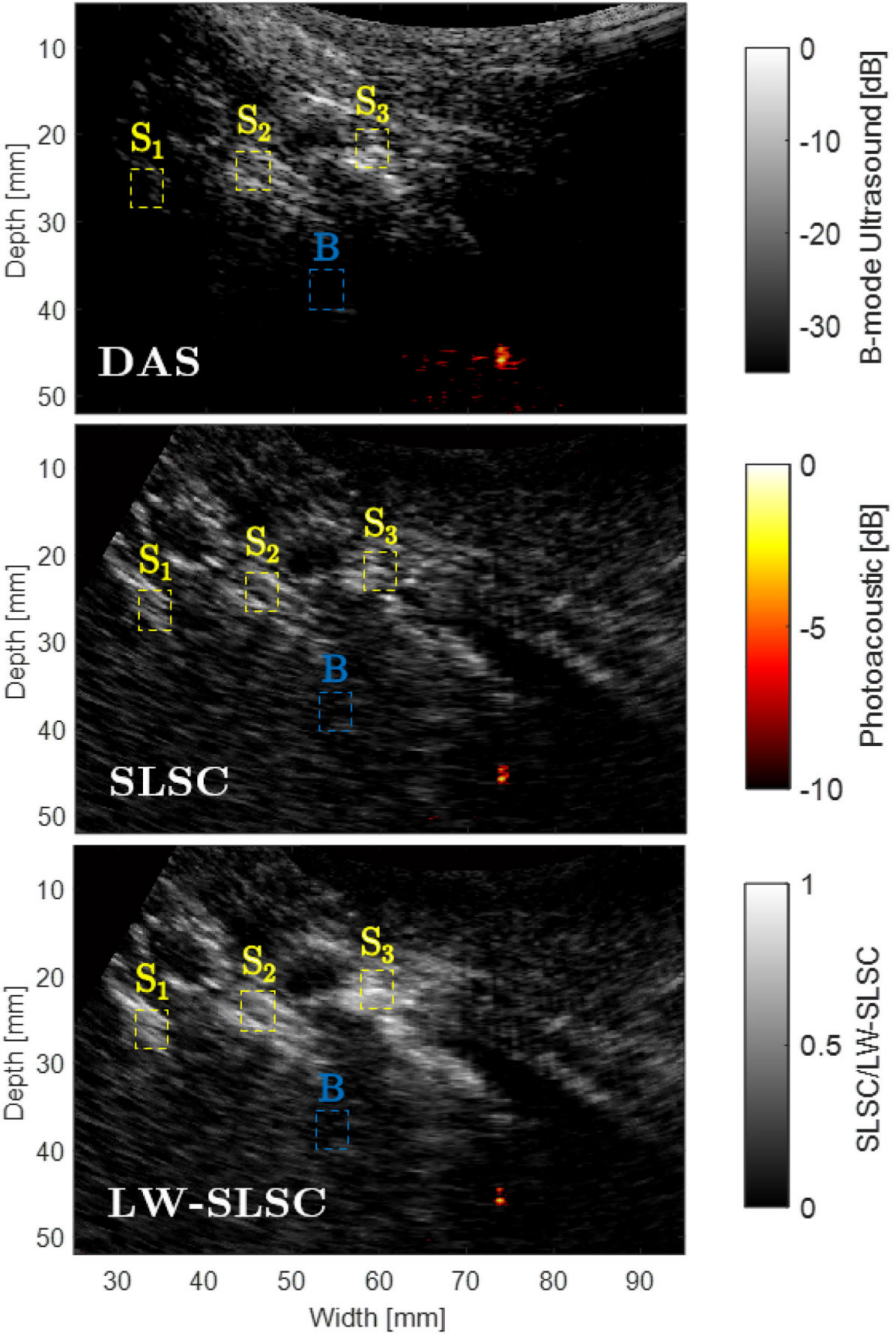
Examples of ultrasound and co-registered photoacoustic images from an oblique sagittal view of L3-L5 vertebrae reconstructed with DAS, SLSC and LW-SLSC. *S*_1_, *S*_2_, *S*_3_, and *B* denote the selected regions for quantitative assessments.
